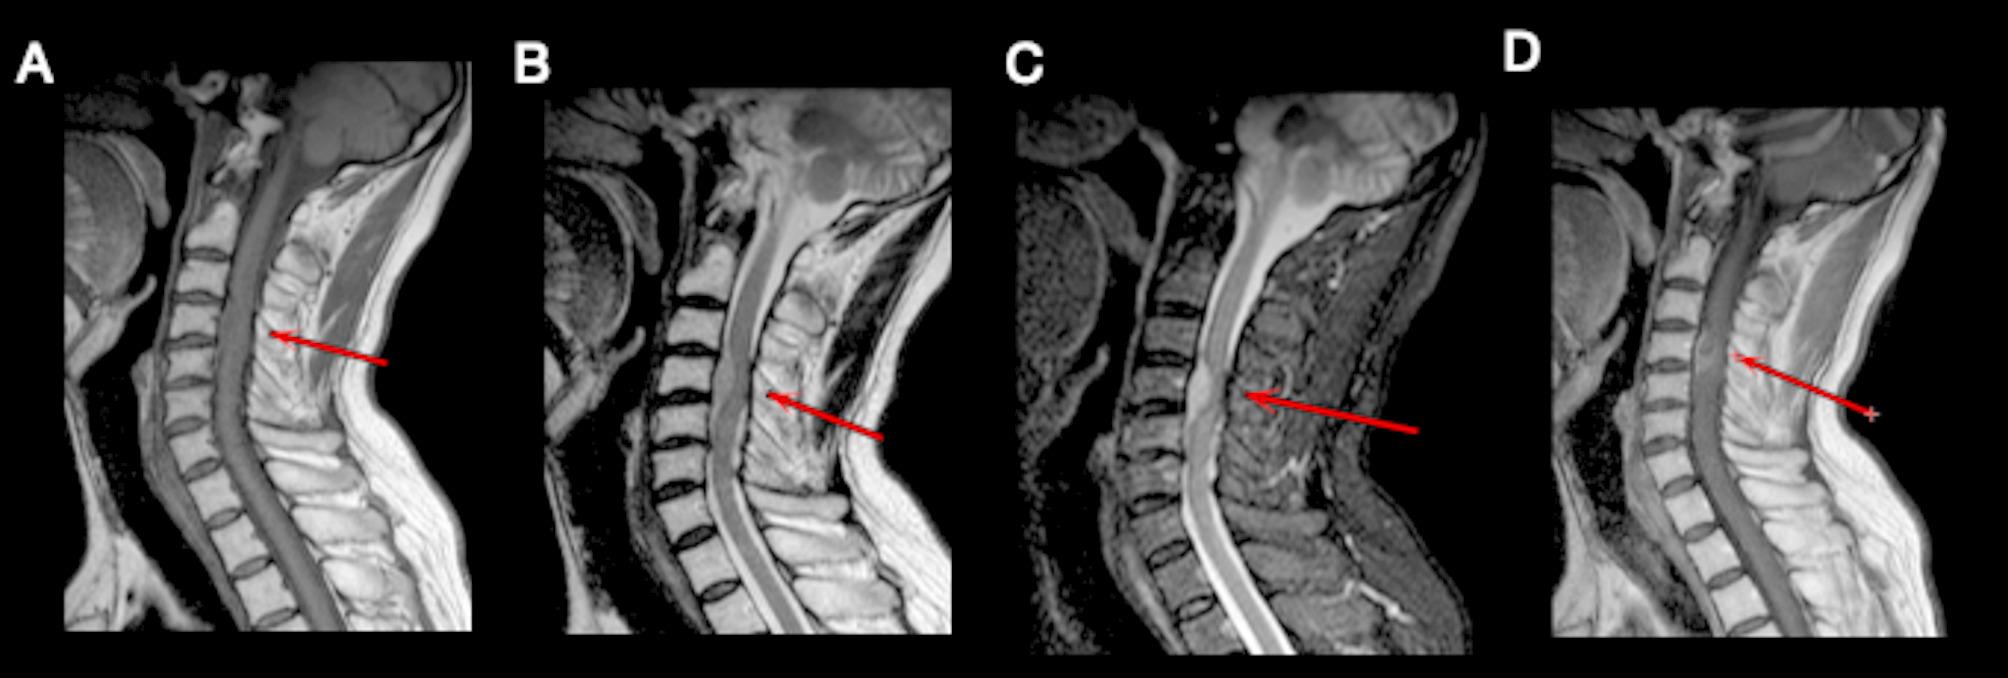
Cervical spine MRI images. (A) Sagittal T_1_W sequence, pre-contrast; (B) sagittal T_2_W sequence; (C) sagittal T_2_-STIR sequence; (D) gadoliniuma focal enhancing lesion at C4 level.
